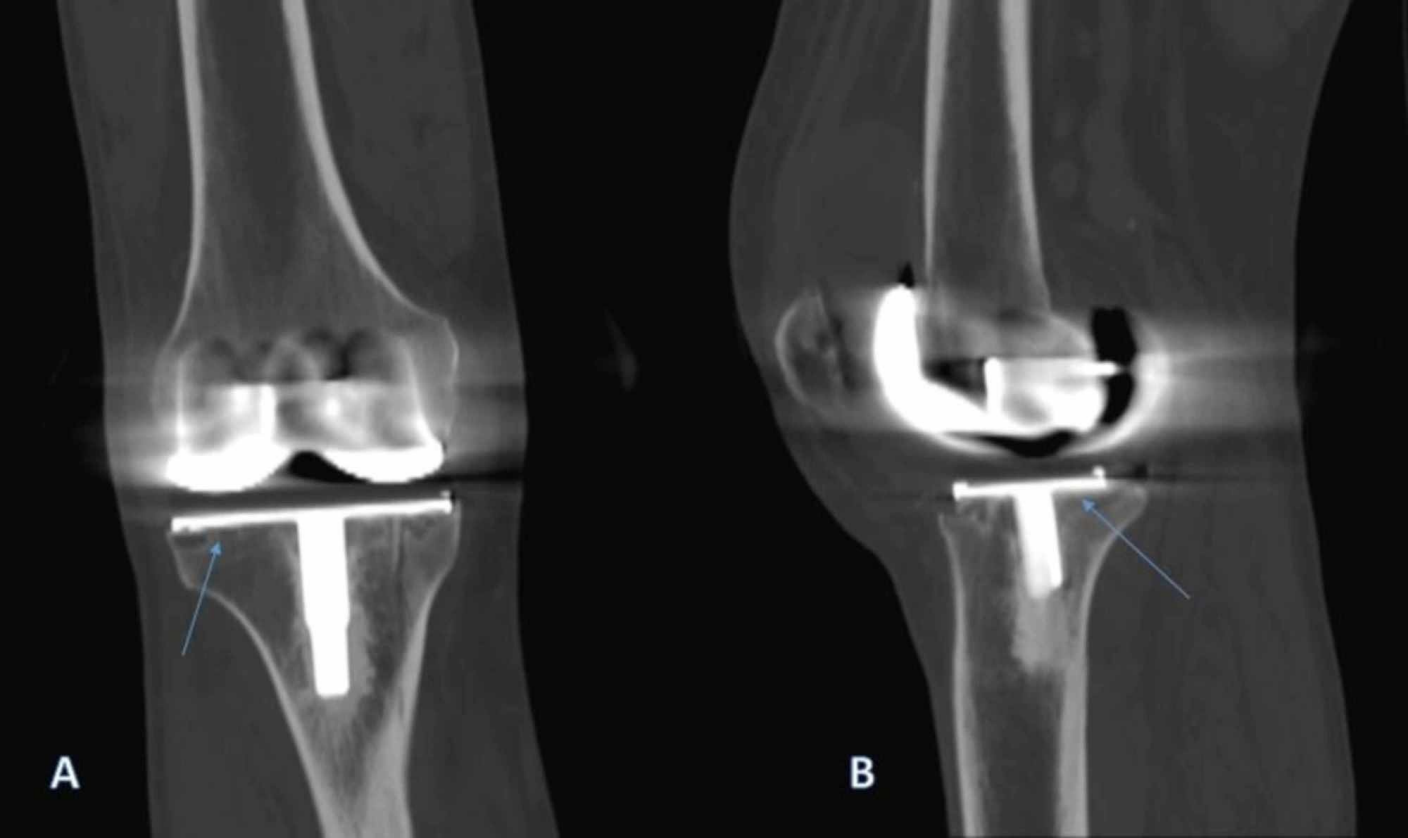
Computed tomography (CT) scan of the right knee Coronal (A) and sagittal (B) views show minimal periprosthetic osteolysis areas underneath the tibial base plate with no obvious loosening of the components
